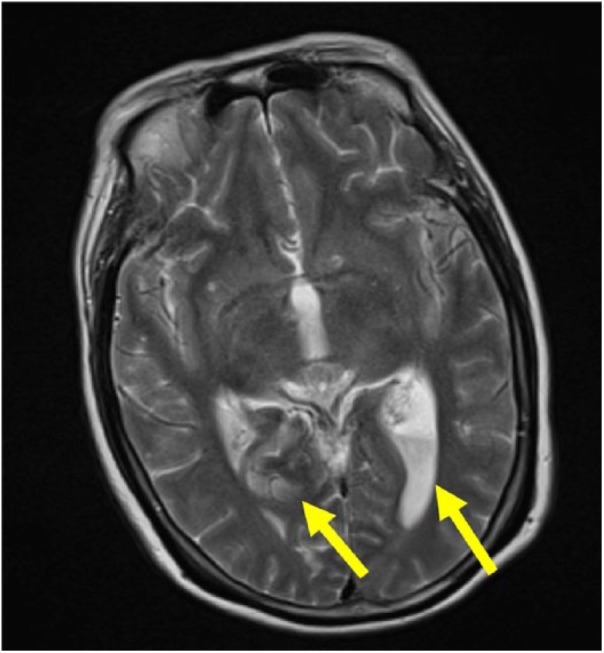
Brain magnetic resonance imaging demonstrating meningitis and ventriculitis. The arrow on the left points to diffuse leptomeningeal enhancement most conspicuous along the bilateral temporal lobes/insula, subarachnoid space of the basal cisterns and ventricular system. The arrow on the right points to additional proteinaceous material/pus within the subarachnoid space and dependently in the bilateral lateral ventricles.
